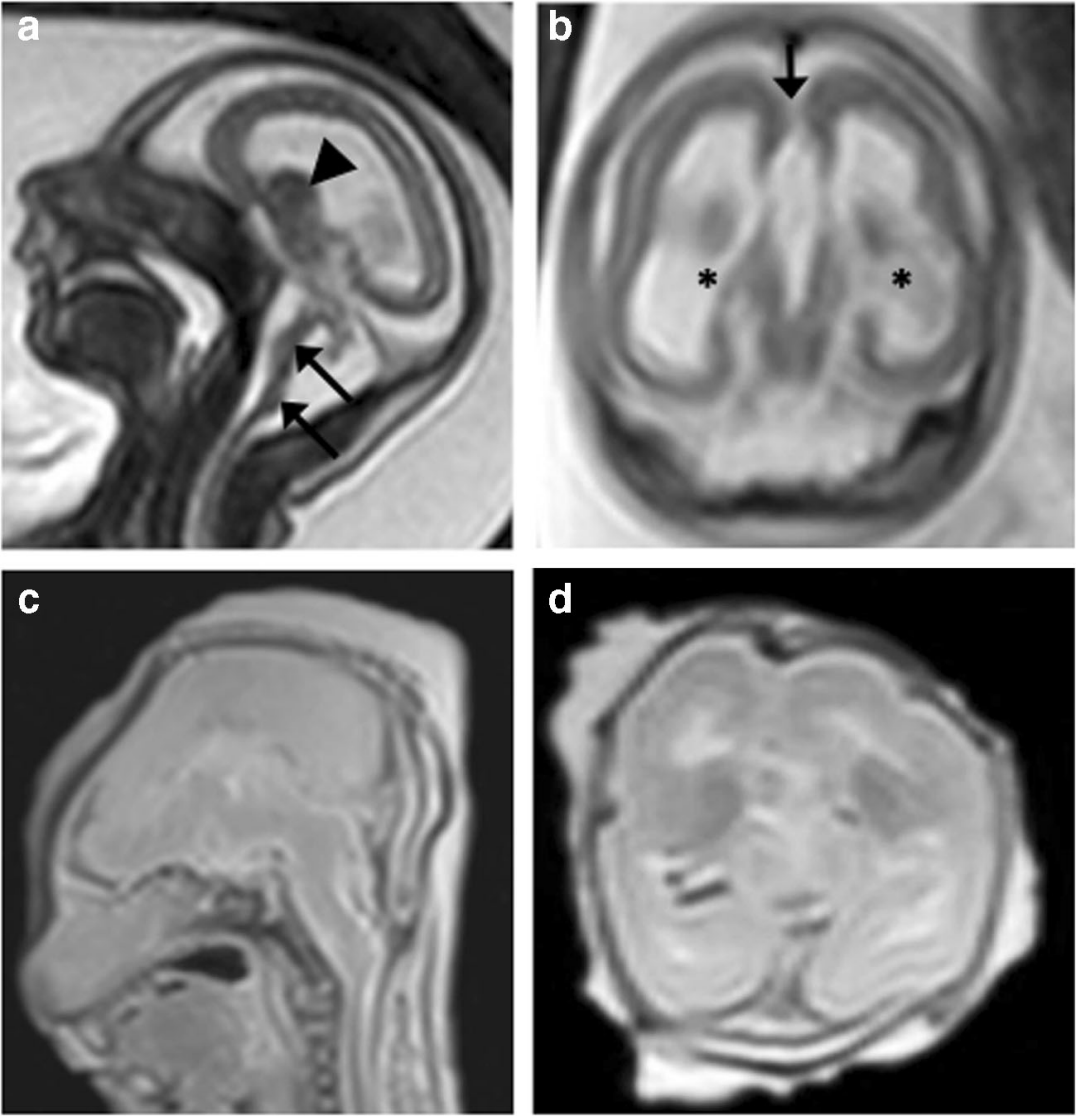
Tubulinopathy in a 23-week gestation fetus. a Axial T2-weighted iuMR demonstrates microcephaly, severely thinned hemispheric parenchyma, thinly kinked brainstem (black arrows), small malformed cerebellum with enlarged ganglionic eminence (arrowhead). b Coronal single shot T2-weighted iuMR image demonstrates bilateral lateral ventriculomegaly (asterisks) and an absent corpus callosum (black arrow). c, d Coronal and axial T2-weighted PMMR images show decompressed lateral ventricles. There is also difficulty in appreciating the intra-uterine cerebellar abnormalities
